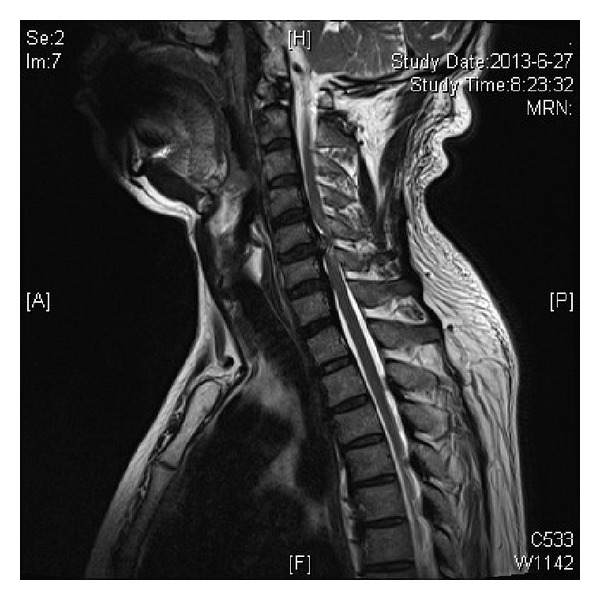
T2-weighted sagittal magnetic resonance image. A C5-6 herniated disc is seen indenting the cord. The mass is surrounded by CSF signal intensity margin, and caudally the ventral dura line appears divided into two, the so-called “Y-sign.”
